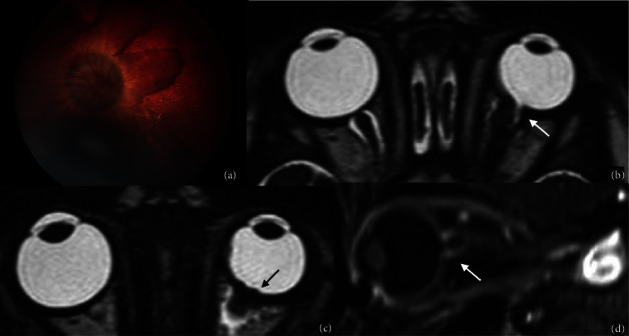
Morning Glory syndrome. RET-cam image in an eighteen-month-old boy with microphthalmia and Morning Glory malformation (a). MRI of the orbits confirms the malformation: axial high-resolution T2WI shows a funnel-shaped optic disc (white arrow in (b)) and the elevation of the retina (black arrow in (c)), whereas a sagittal postcontrast T1WI shows a discontinuous sclera profile (white arrow in (d)).
